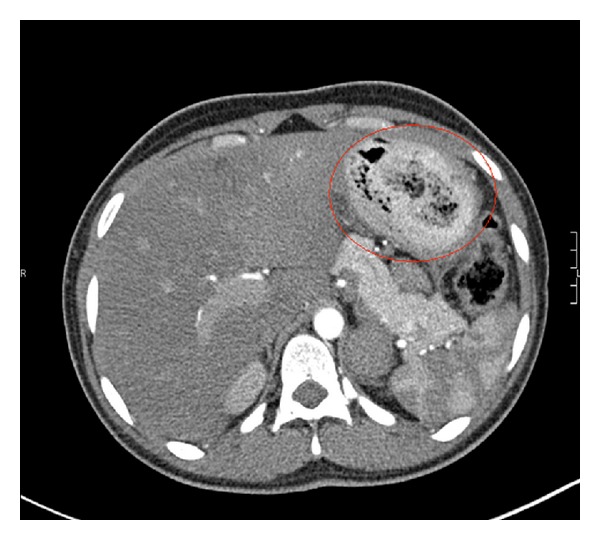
Computed tomography of the abdomen showing a trichobezoar located in the stomach (circled in red).
